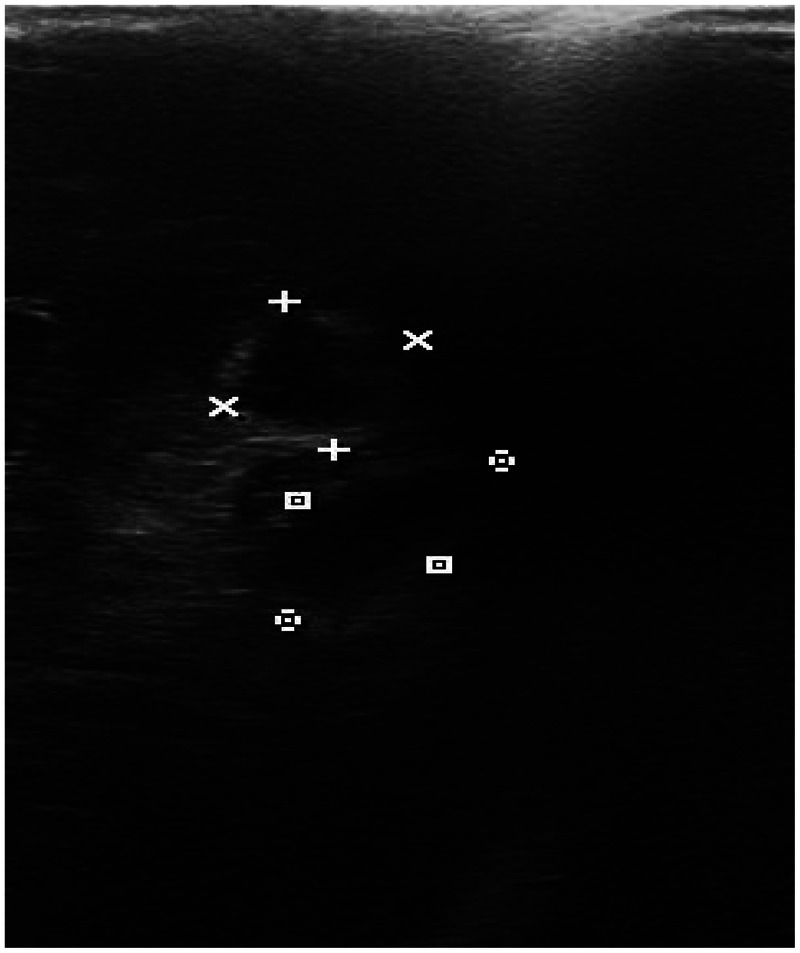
Cranial ultrasound of patient 7 revealed two abnormal echoes in the left occipital lobe parenchyma, measuring approximately 11 × 10 mm and 15 × 8 mm, respectively. The lesions exhibited relatively well-defined margins, were surrounded by a slightly hyperechoic rim, and showed reduced internal echogenicity.
